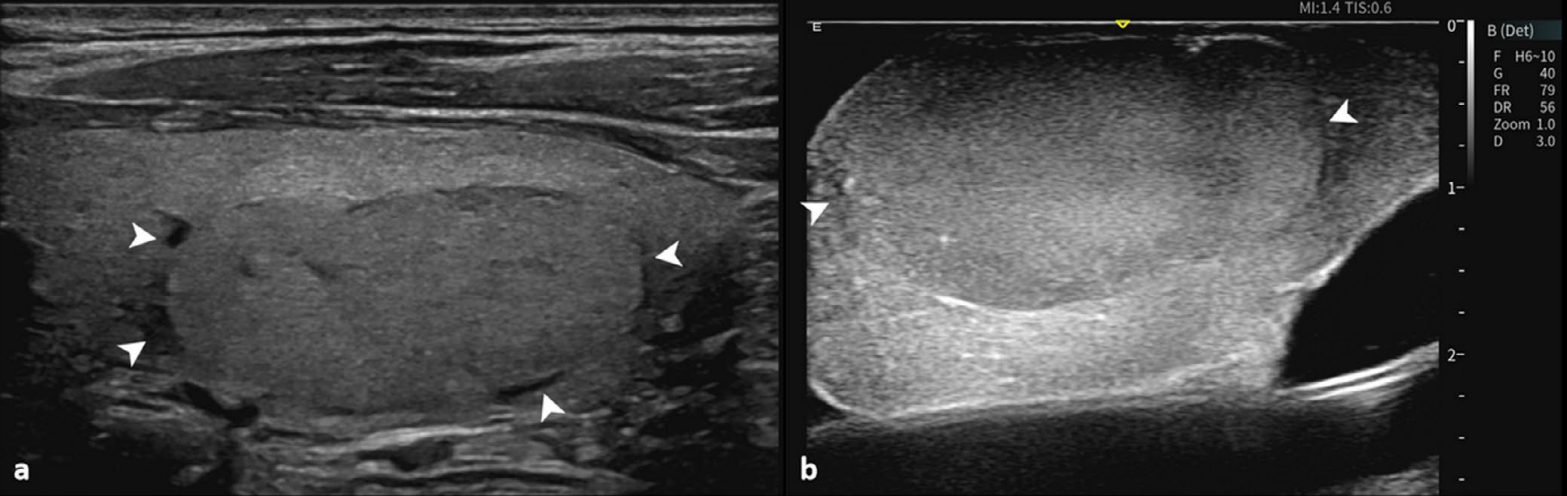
Comparison of thickness of the nodule's halo before and after nodule resection. (a) Conventional ultrasound image reveals an uneven halo of the nodule (arrows). (b) Intraoperative ultrasound demonstrates a reduction in thickness of the nodule's halo (arrows), following the resection of the thyroid lobe. The attenuation of the halo's thickness is attributed to the blockage of the thyroid arteries.
